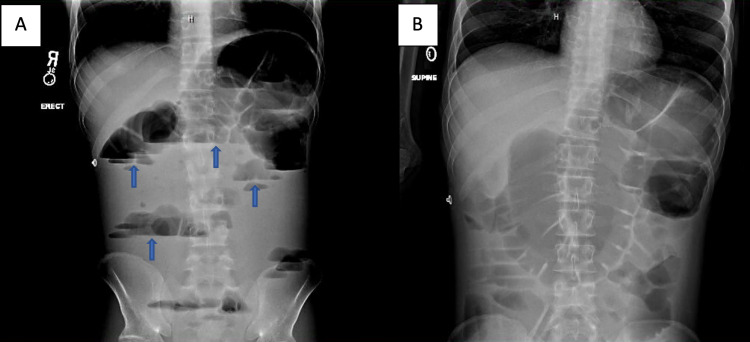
Abdominal anterior-posterior X-ray film. (A) and (B) demonstrate signs of small bowel obstruction with extensive dilations of the bowel and numerous air-fluid levels (marked by blue arrows). No signs of air are appreciated in the large intestine.
